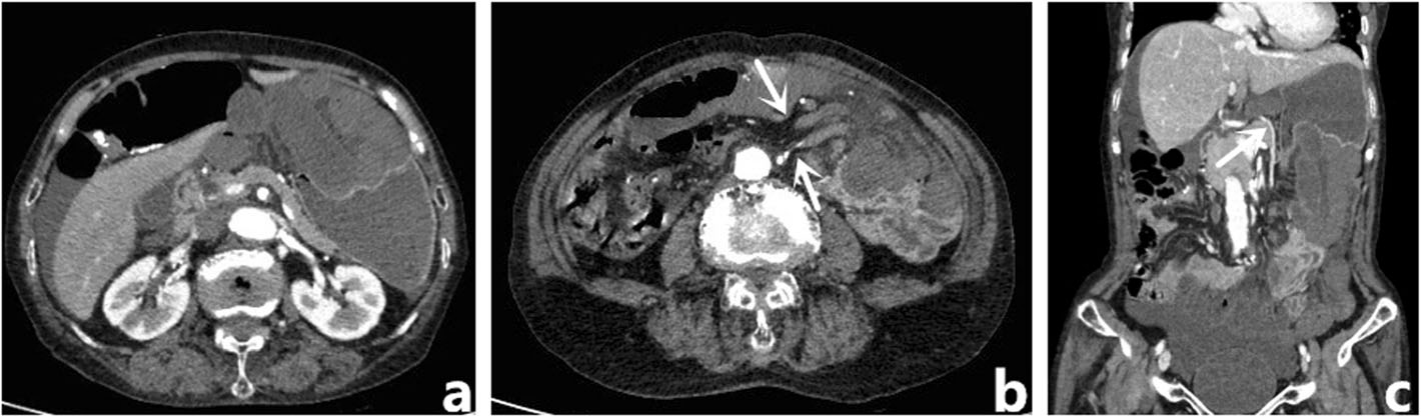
Abdominal CT images. a There is distention of the small intestine in the distal, ventral part of the distorted stomach, accumulated liquid around the liver; and reduced enhancement in the intestinal mucosa; (b) The small intestine is prolapsed through the defected lesser omentum, as denoted by the arrows; (c) These is dislocation of the blood vessels of the small intestine and distorted stomach, as indicated by arrow
